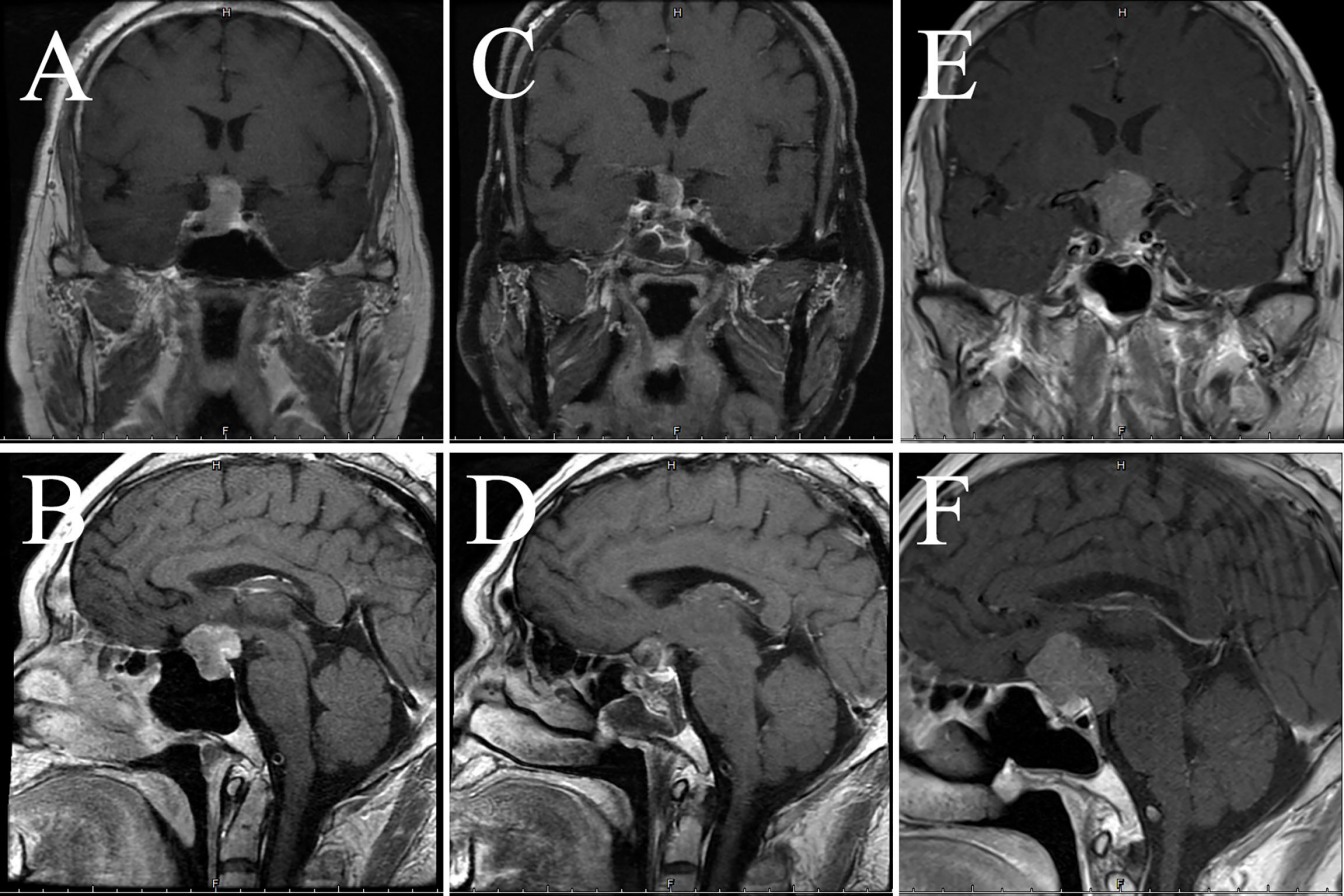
Imaging data of case 2. The images show coronal and sagittal MRI of the patient’s head preoperatively (A, B), three months postoperatively (C, D), and twenty-one months postoperatively (E, F), respectively.
